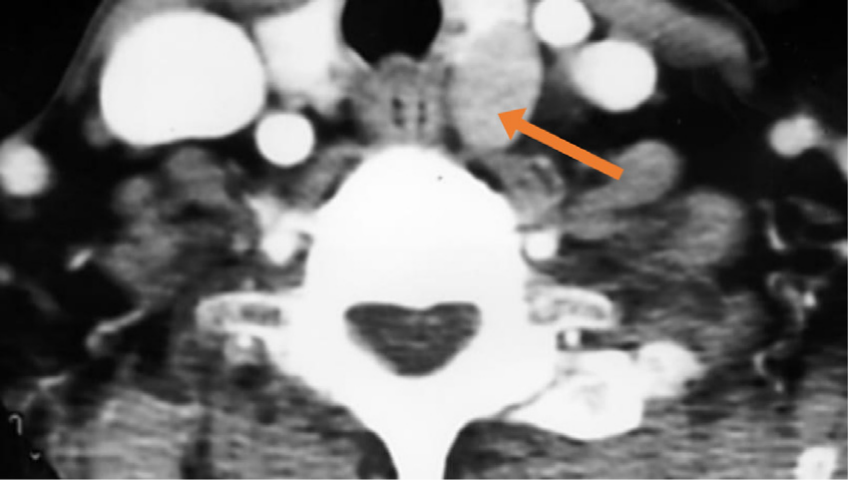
Contrast CT scan of the neck showing a parathyroid adenoma posterior to the left lobe of the thyroid.
